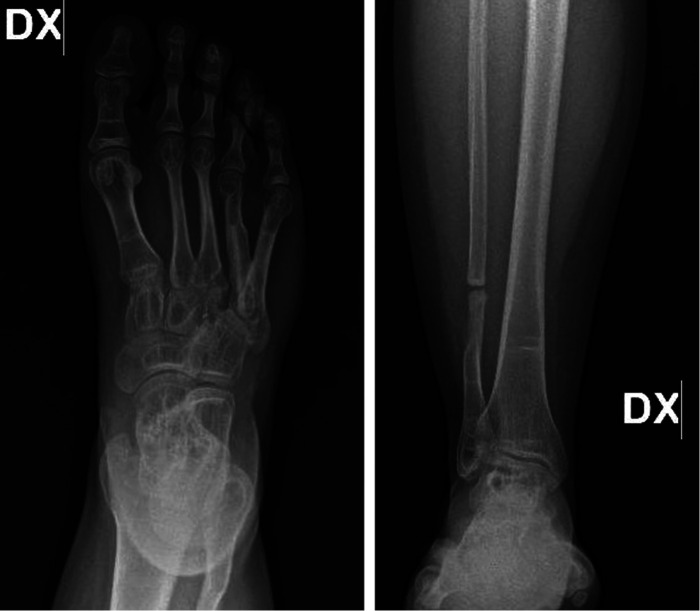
Follow-up x-ray of the foot and leg respectively taken at 12 months (A) and 24 months (B) showing incorporation of graft with no evidence of recurrences or other lesions and the donor site with an almost full recovery of the bone fibular gap.
